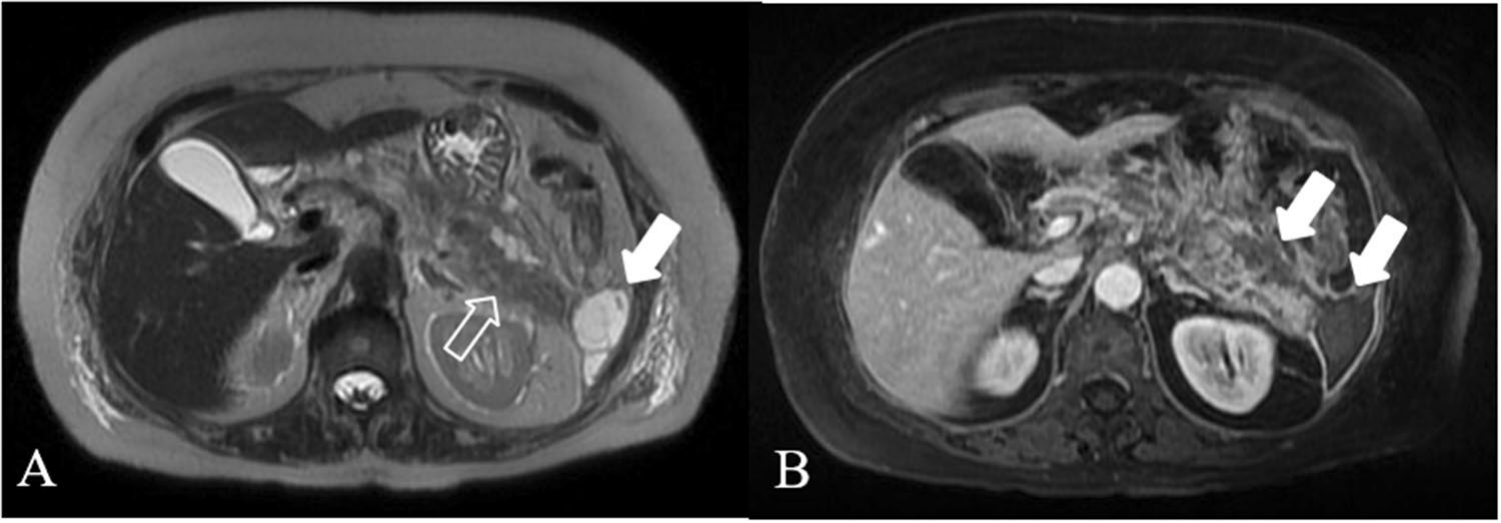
Walled off necrosis. 73 years old woman undergoing statins and amiodarone treatment. T2 weighted MR image (a) and post-gadolinium T1w scan (b), show the presence of a heterogeneous encapsulated fluid collection suggesting walled-off necrosis (WON); the WON extends in the pancreatic and peripancreatic area, up to the left anterior pararenal space and left paracolic space (arrows). Diffuse hyperintensity on T2w images of the pancreatic parenchyma indicates the presence of parenchymal edema (empty arrow in a)
